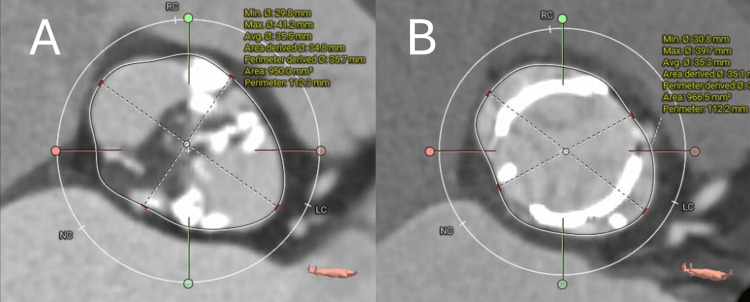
Pre- and postoperative CT curved planar reconstruction (CPR) images at the annular level. (A) Preoperative image shows an elliptical-shaped annulus characteristic of Type 0 bicuspid aortic valve. (B) Postoperative image demonstrates a more circular expansion of the annulus achieved with the hybrid suture technique, addressing the anatomical challenge that predisposes to paravalvular leakage.
